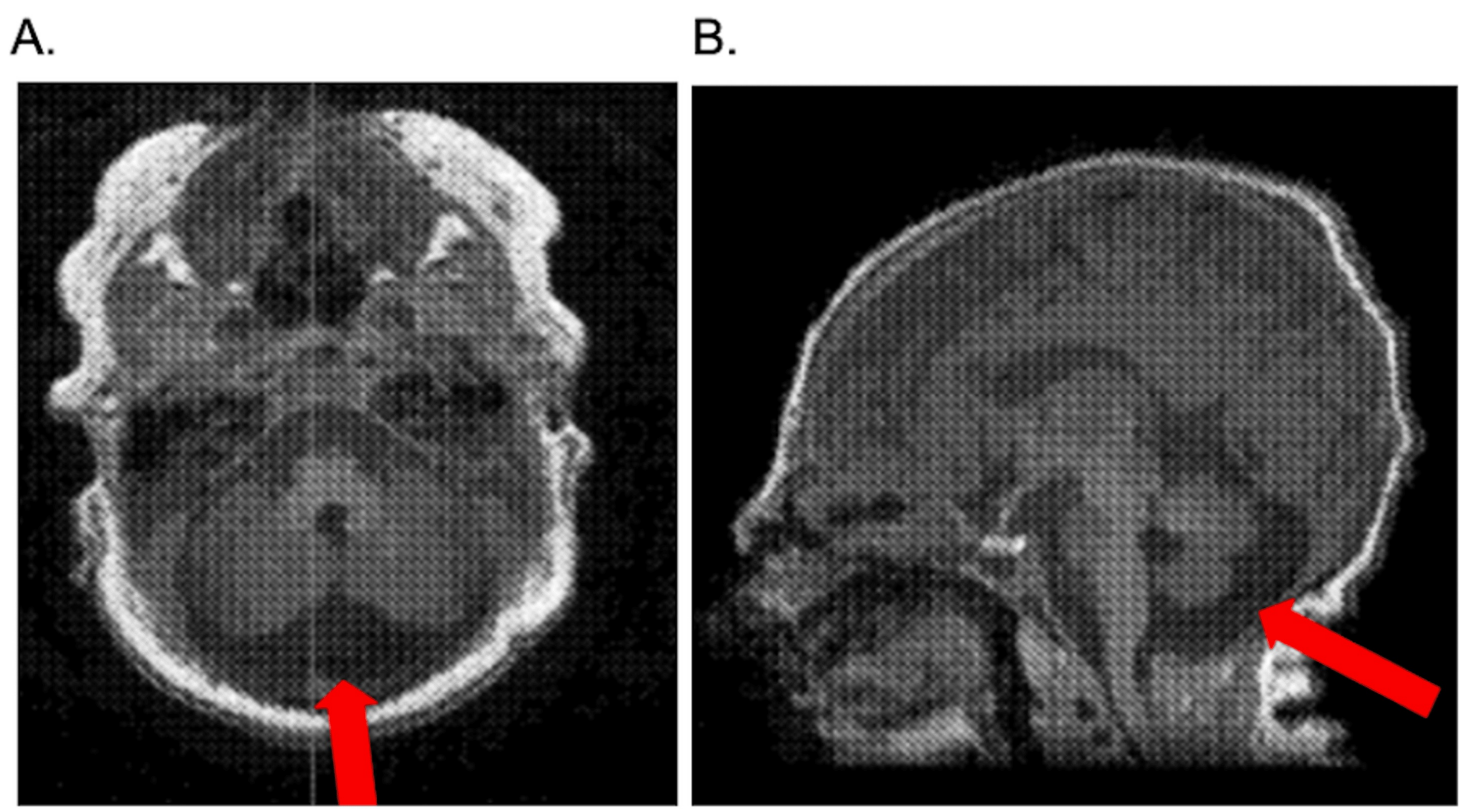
Brain MRI without contrast demonstrating mild prominence of the posterior fossa cerebrospinal fluid. (A) Axial view. (B) Sagittal view.
